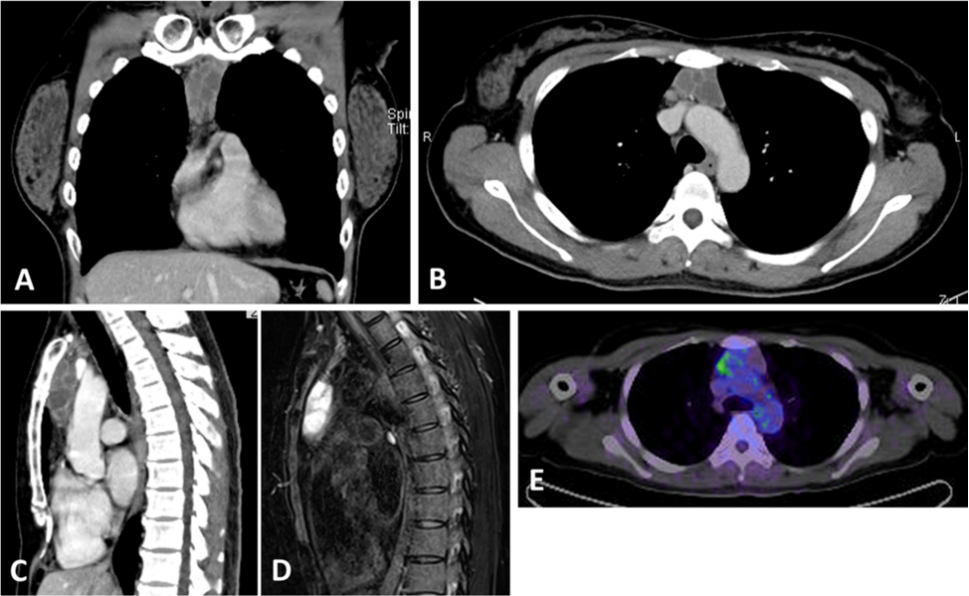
Radiological imaging of the lesion. Contrast-enhancement computed tomography (CT) shows a tumor in the superior anterior mediastinum with slightly enhanced multiple septa (a-c). Magnetic resonance imaging (MRI) with fat suppression reveals the tumor with high-intensity (d). The standardized uptake value (SUVmax) of positron emission tomography (PET)-CT was 4.25, which was relatively low (e)
